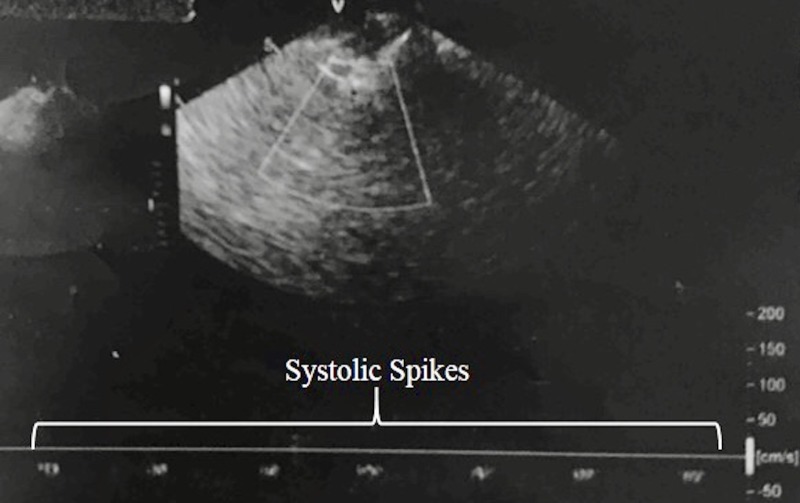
Transcranial Doppler (TCD). TCD showing systolic spikes in the anterior circulation.
